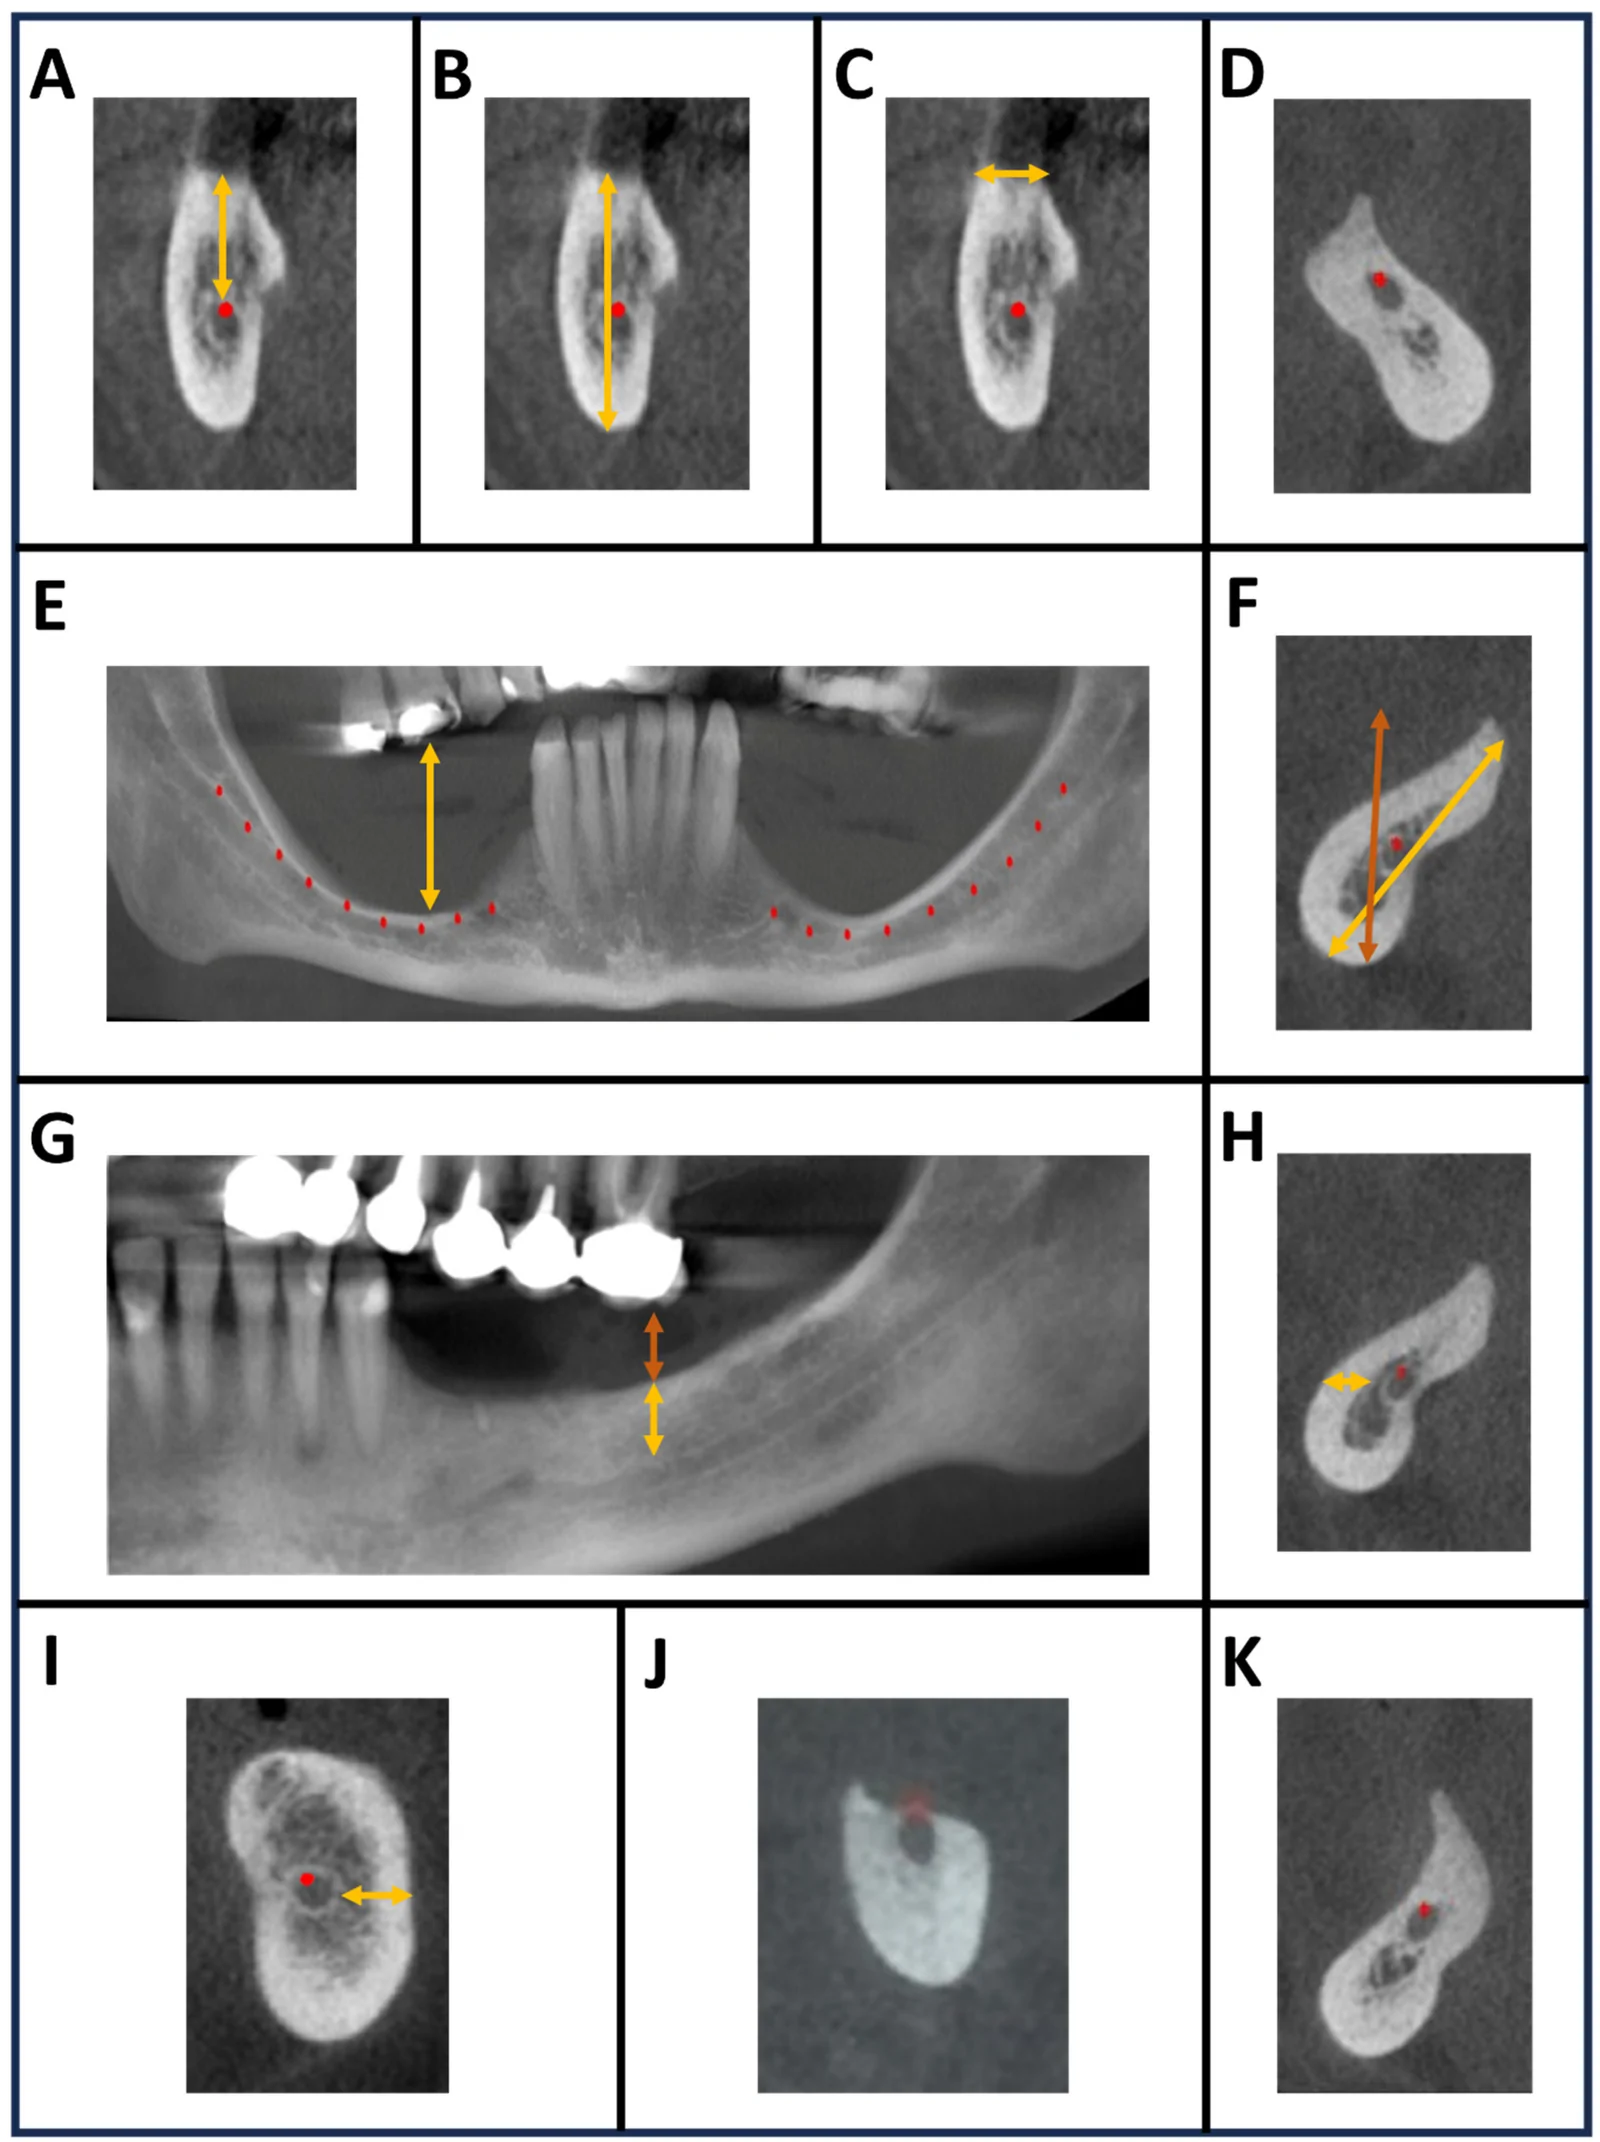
Key radiographic parameters guiding preoperative planning for inferior alveolar nerve repositioning. (A) Crest-to-canal height; (B) global mandibular height; (C) buccolingual crestal width; (D) bone density evaluation (D1); (E) excessive intermaxillary space; (F) ridge inclination relative to the desired implant axis; (G) reduced intermaxillary space; (H) buccal cortical plate thickness relevant to lateral window design; (I) lingual position of the mandibular canal; (J) high vertical course of the canal; (K) knife-edge ridge requiring alveoloplasty.
